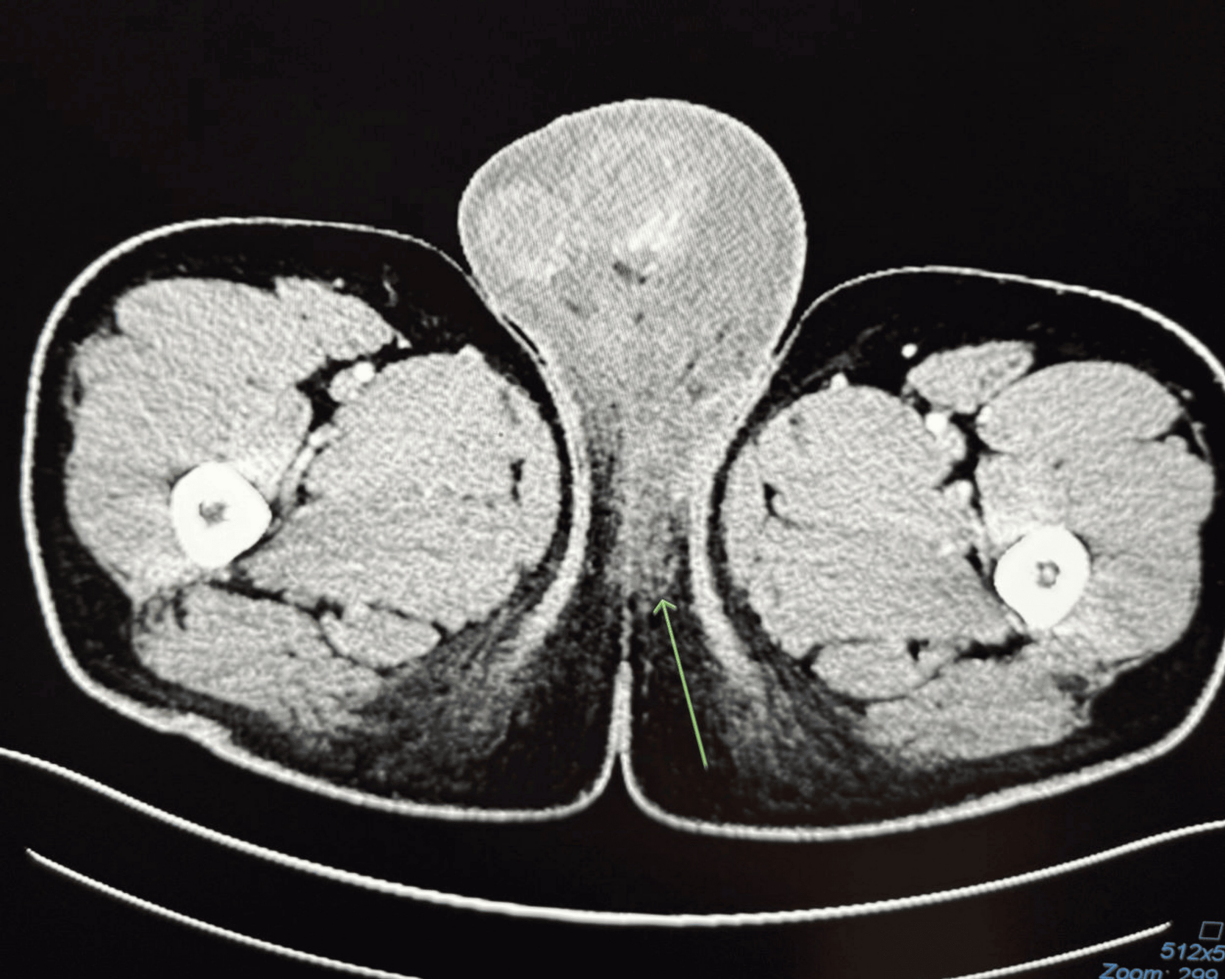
Computed tomography of abdomen and pelvis with contrast: pronounced scrotal swelling, left greater than right, with inflammatory changes tracking into groins (green arrow); no drainable abscess in the image area.
